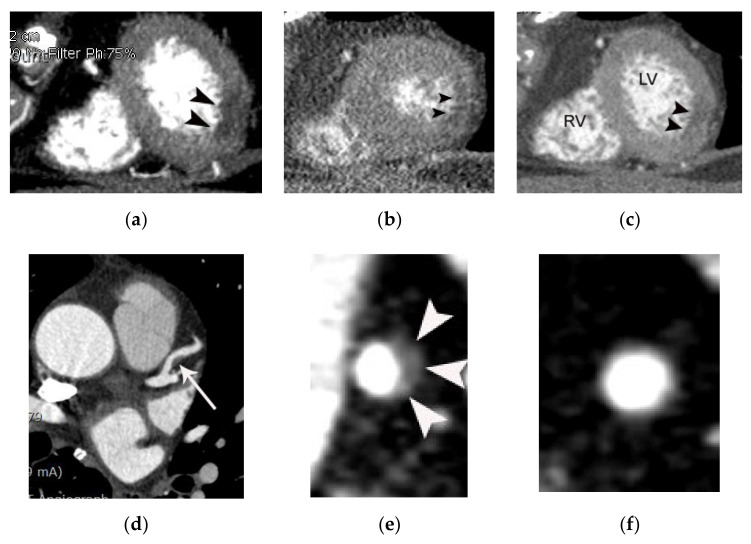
A case of myocardial infarction with nonobstructive coronary arteries (MINOCA) in a 66-year-old female patient. The patient presented to the emergency department (ED) with acute chest pain. Focal hypoattenuation (arrowheads in (a)) in the lateral wall of the apex of the left ventricle is noted on a CT short-axis view. There is akinesia in the same location on a short-axis systolic image (b). Note the absence of wall thickening in this area on the systolic image compared to the diastolic image (b,c). However, the coronary arteries were normal other than nonobstructive coronary stenosis (arrow in (d)) with noncalcified plaque and positive remodeling in the proximal left anterior descending coronary artery on an axial coronary CT image (d). Note the noncalcified plaque with positive remodeling on an en face view (arrowheads in (e)) compared to the normal reference level (f). Subsequent coronary angiography confirms the CT findings (not shown). RV and LV indicate right and left ventricle, respectively.
